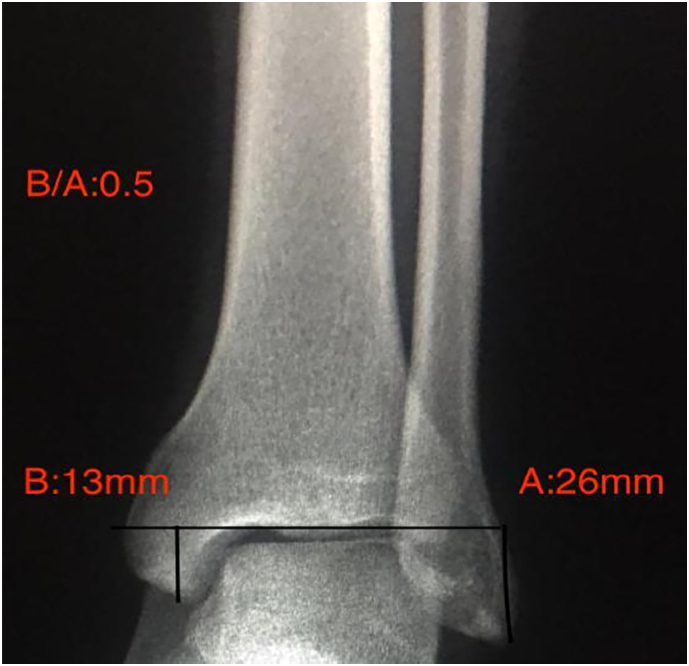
Frontal radiograph shows hypoplasia of the medial malleolus assessed with the ratio between the length of the malleolus B (medial) and A (lateral) according to the method of Elisé et al.
